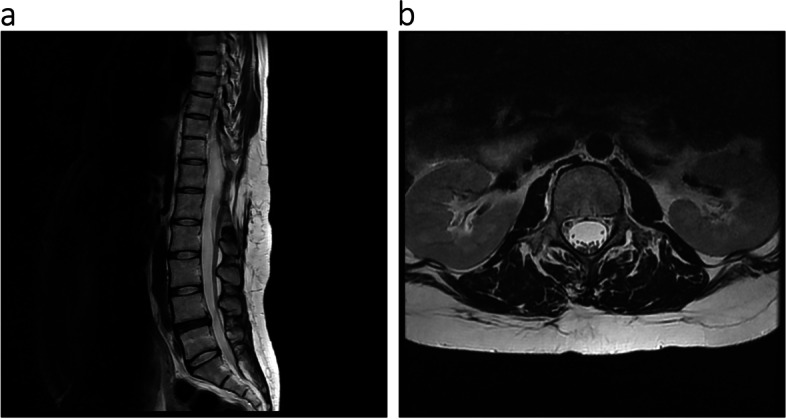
Magnetic resonance imaging for pregnant woman with a history of spina bifida. a Sagittal MRI imaging revealed postoperative change at T12/L1 intervertebral space. Epidural space was not apparent from T11 to L2 but became apparent below L2. b Both anesthesiologists and orthopedic surgeons confirmed the L3/4 intervertebral epidural space
